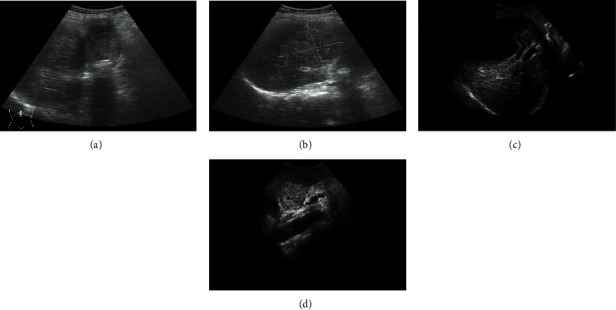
Contrast-enhanced ultrasound examination. (a) The patient was a 62-year-old male with a history of gallbladder stones for 5 years and epigastric pain for 5 days. Contrast-enhanced ultrasound examination of the abdomen showed that the continuity of the gallbladder wall was locally interrupted, and a slightly strong echogenic nodule protruding into the cavity was seen near the bottom. (b) The patient was a 55-year-old female with dull pain in the upper abdomen for half a year. Contrast-enhanced ultrasound examination of the abdomen showed the thickening of the gallbladder wall, and a moderate echogenic mass filled the cyst cavity at the bottom of the gallbladder. (c) The patient was a 53-year-old female with abdominal pain for 3 days. Contrast-enhanced ultrasound examination of the abdomen showed that the gallbladder volume increased significantly, and a solid mass with irregular shape was seen in the cyst cavity. (d) The patient was a 64-year-old female with a history of gallbladder stones for 5 years and epigastric pain for 5 days. Contrast-enhanced ultrasound examination of the abdomen showed clear boundaries of gallbladder lesions and irregular thickening of the cyst wall.
